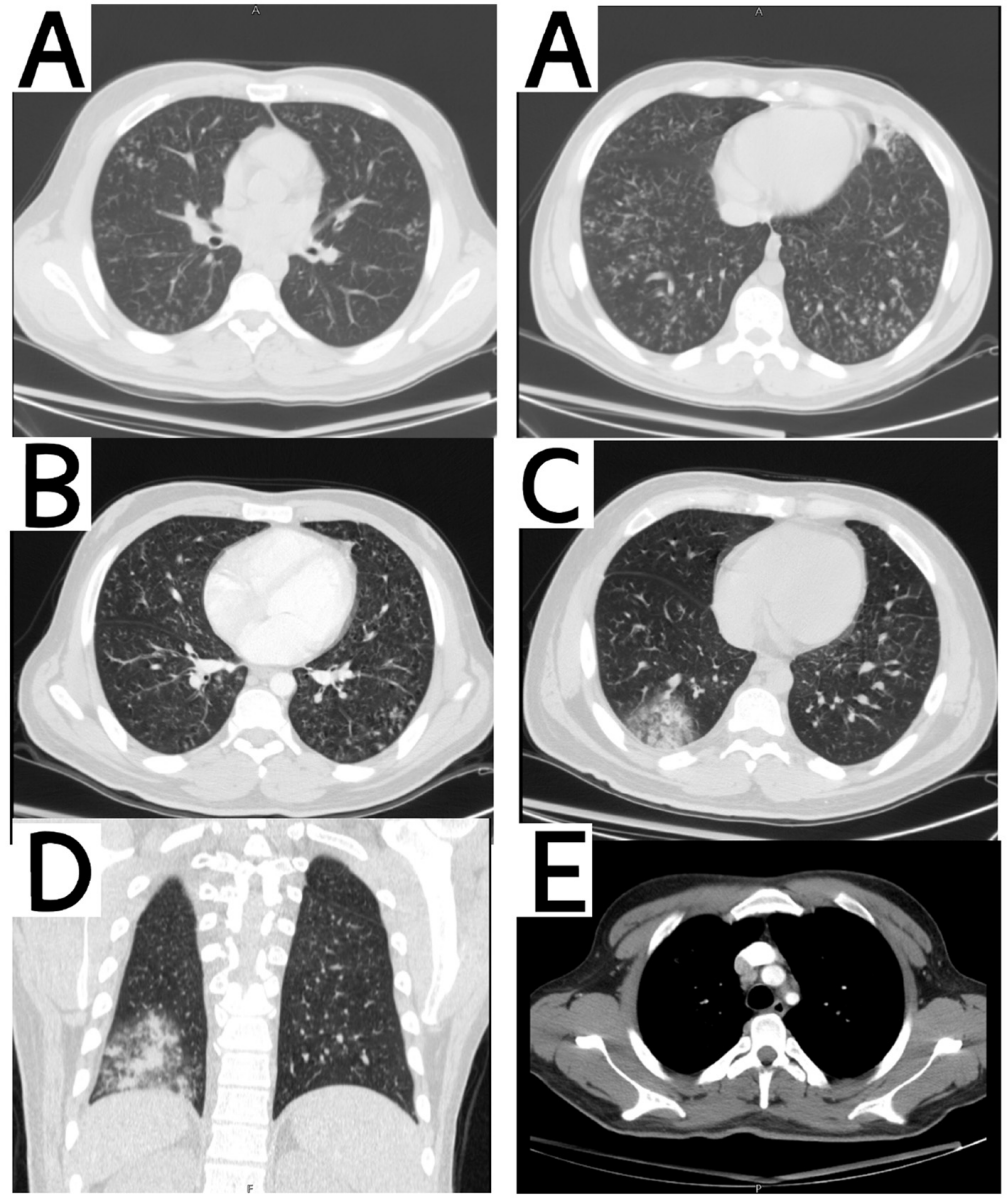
(A) Axial chest CT showing miliary nodules and tree-in-bud pattern. (B) Axial chest CT following anti-tubercular therapy demonstrating no significant radiological improvement. (C) Axial chest CT showing multiple pulmonary opacities, predominantly in the right lower lung zone. (D) Coronal chest CT demonstrating multiple opacities, most prominent in the right lower lung zone. (E) Mediastinal window revealing enlarged lymph nodes.
